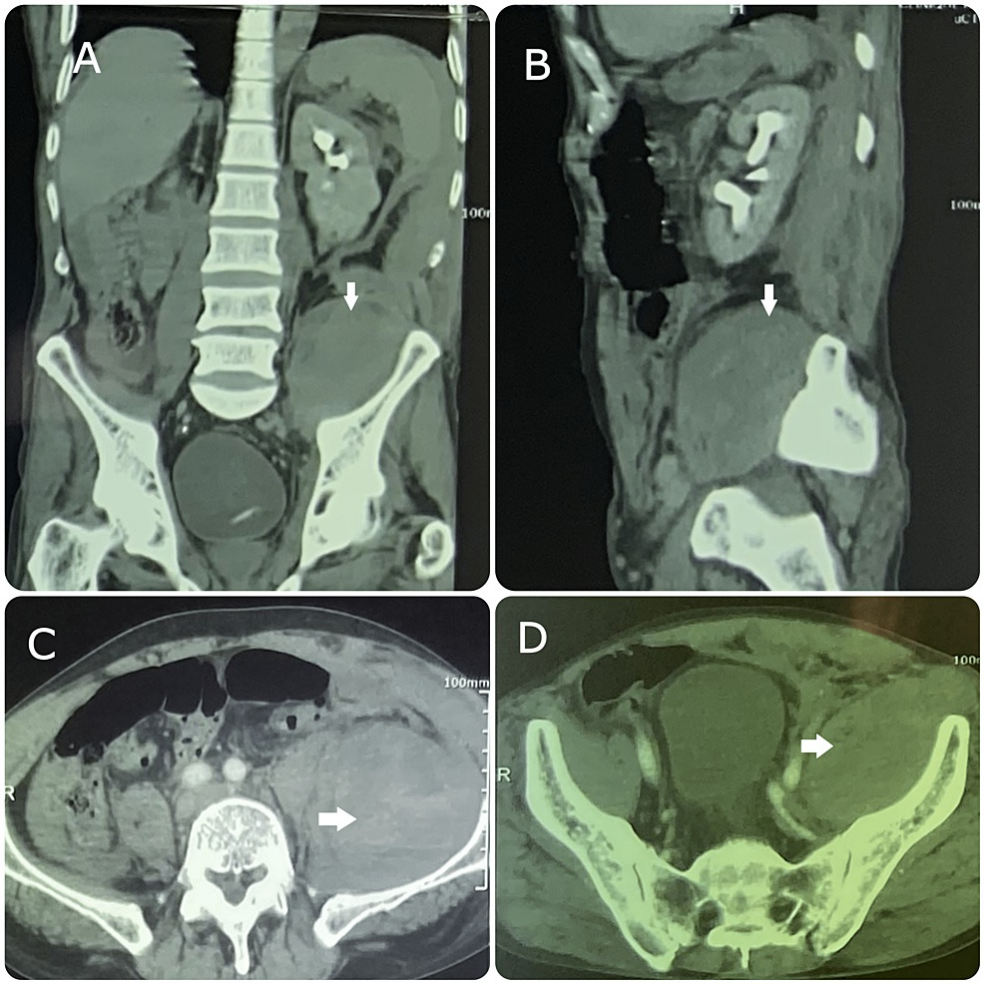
A. Coronal, B. Sagittal, and C and D. Axial abdominal computed tomography showing a large left iliopsoas muscle haematoma (arrows).
